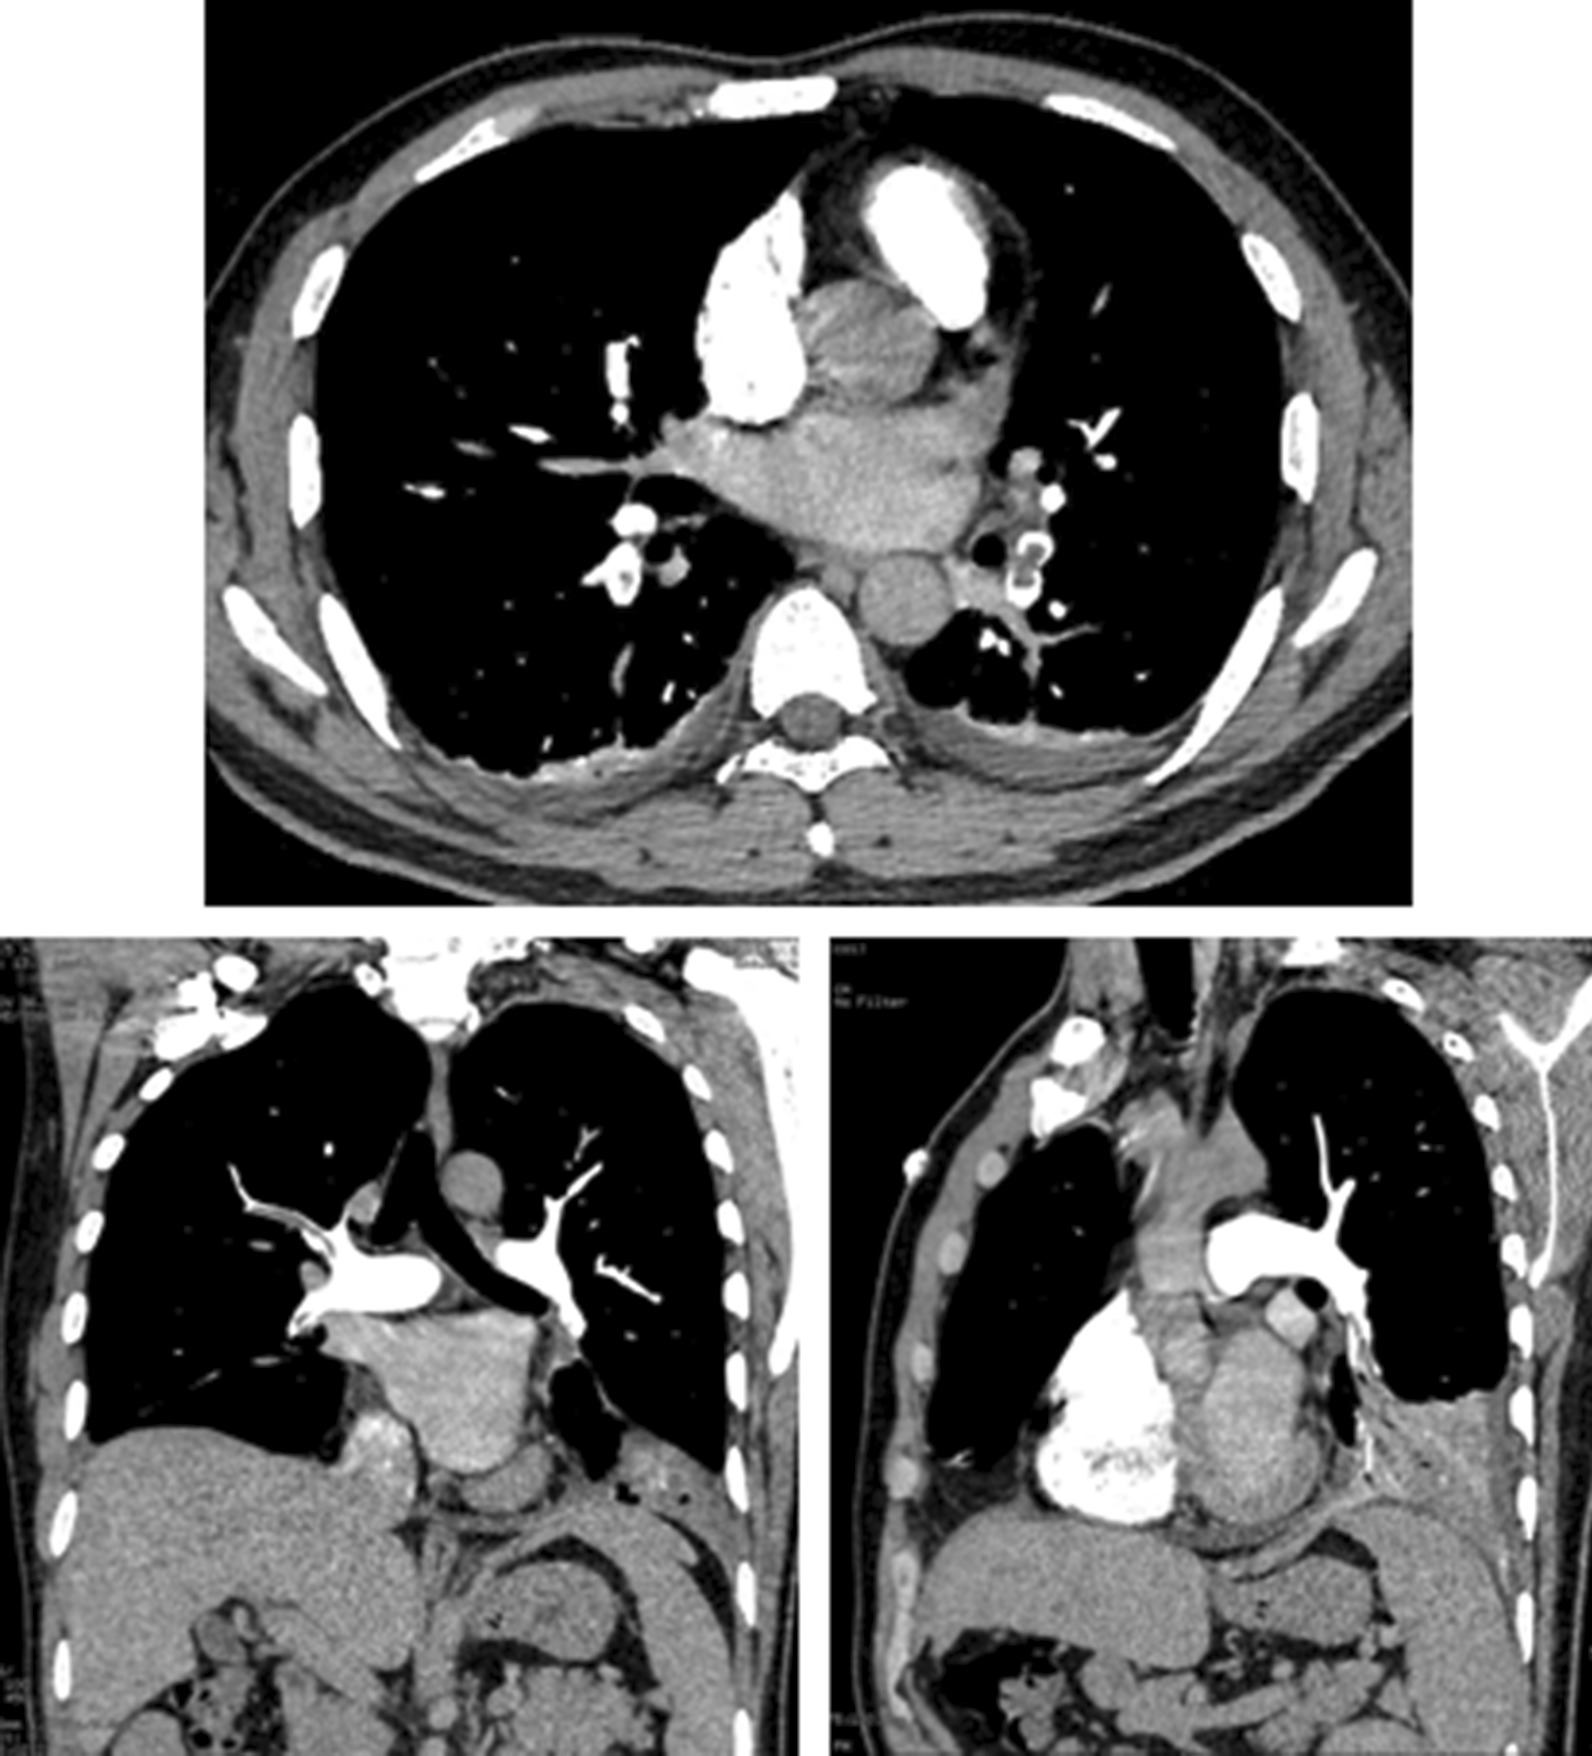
CT-pulmonary artery angiography: bilateral pulmonary embolism of the secondary pulmonary artery and its far branch
